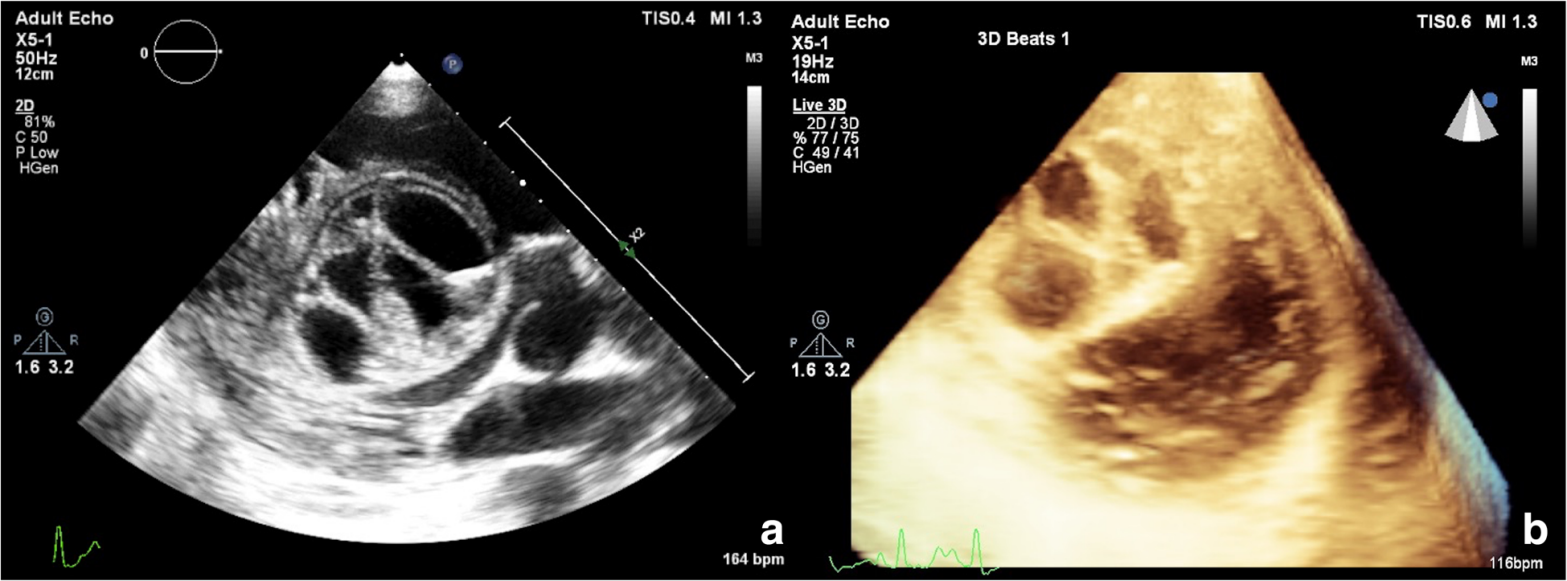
a 2D Echocardiographic and b 3D echocardiographic image showing a multilocular cystic mass over the interventricular septum
